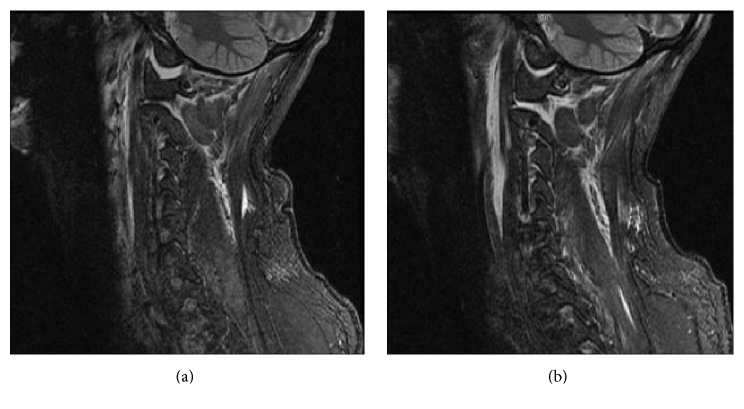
Left and right parasagittal STIR weighted MRI showing displaced occiput-C1 joints.
